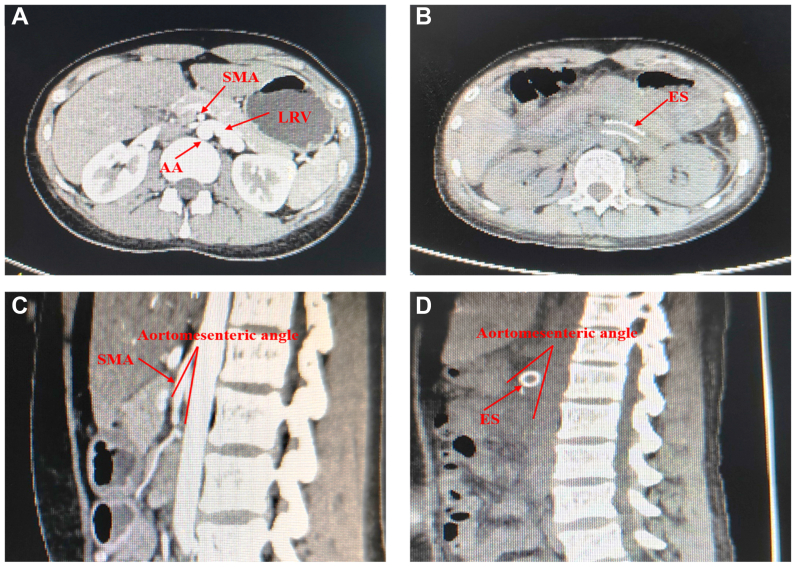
Preoperative and postoperative computed tomography (CT) views of nutcracker syndrome (NCS). (A) (C) Preoperative compression of the left renal vein (*LRV*) between the abdominal aorta (*AA*) and the superior mesenteric artery (*SMA*). (B) (D) Postoperative placement of the extravascular stent (*ES*).
